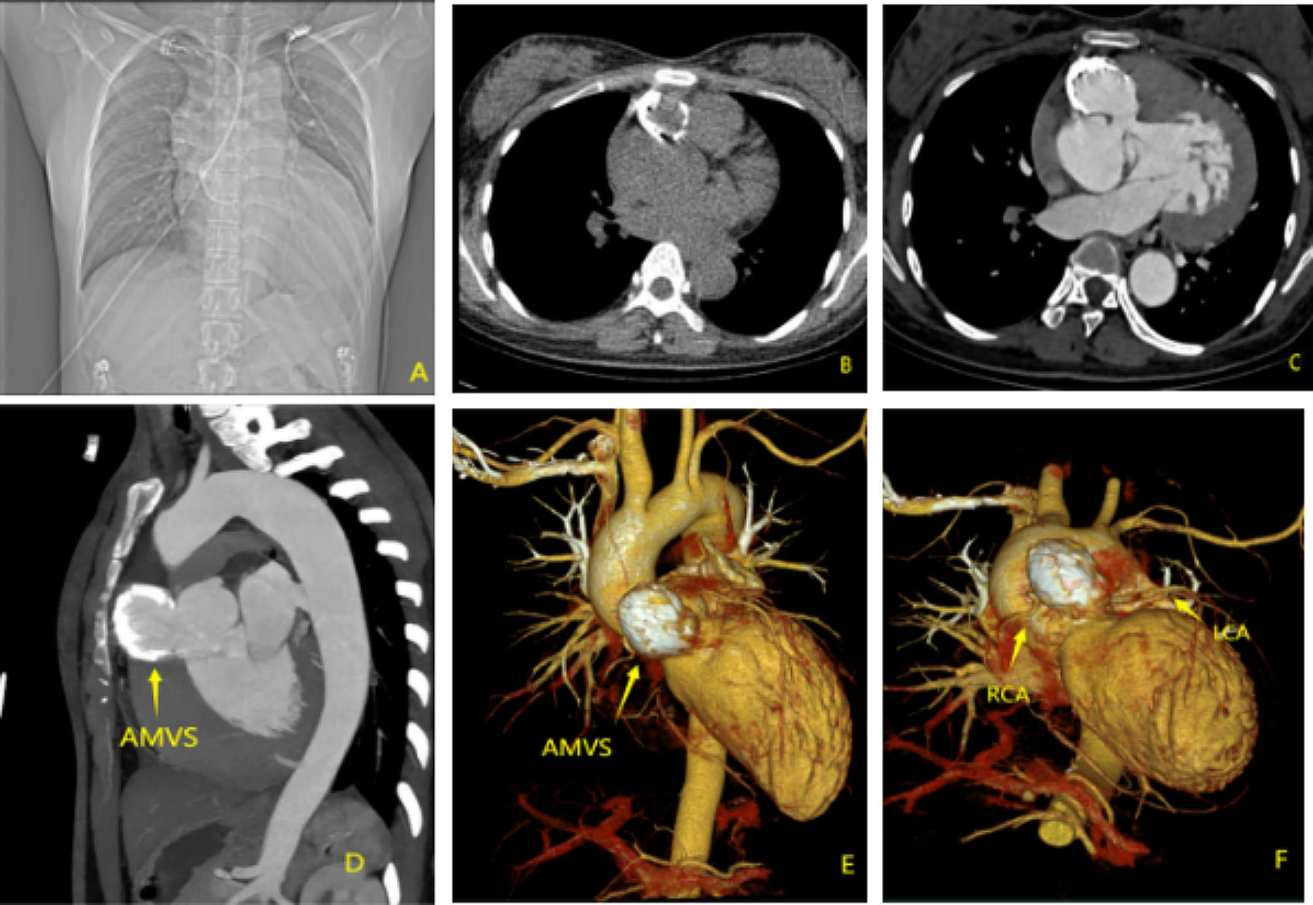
(A) Conventional chest x-ray showed significant enlargement of the left heart and widening of the aortic root. (B) CT showed widespread calcification of the aneurysm wall. C, D Contrast-enhanced CT displayed that the aneurysm was filled with contrast agents and connected to the left ventricular outflow tract. E. Three-dimensional CT showed a dilated ascending aorta with a giant aneurysm located below the aortic root. F. CTA showed the aneurysm was located close to the right coronary artery
